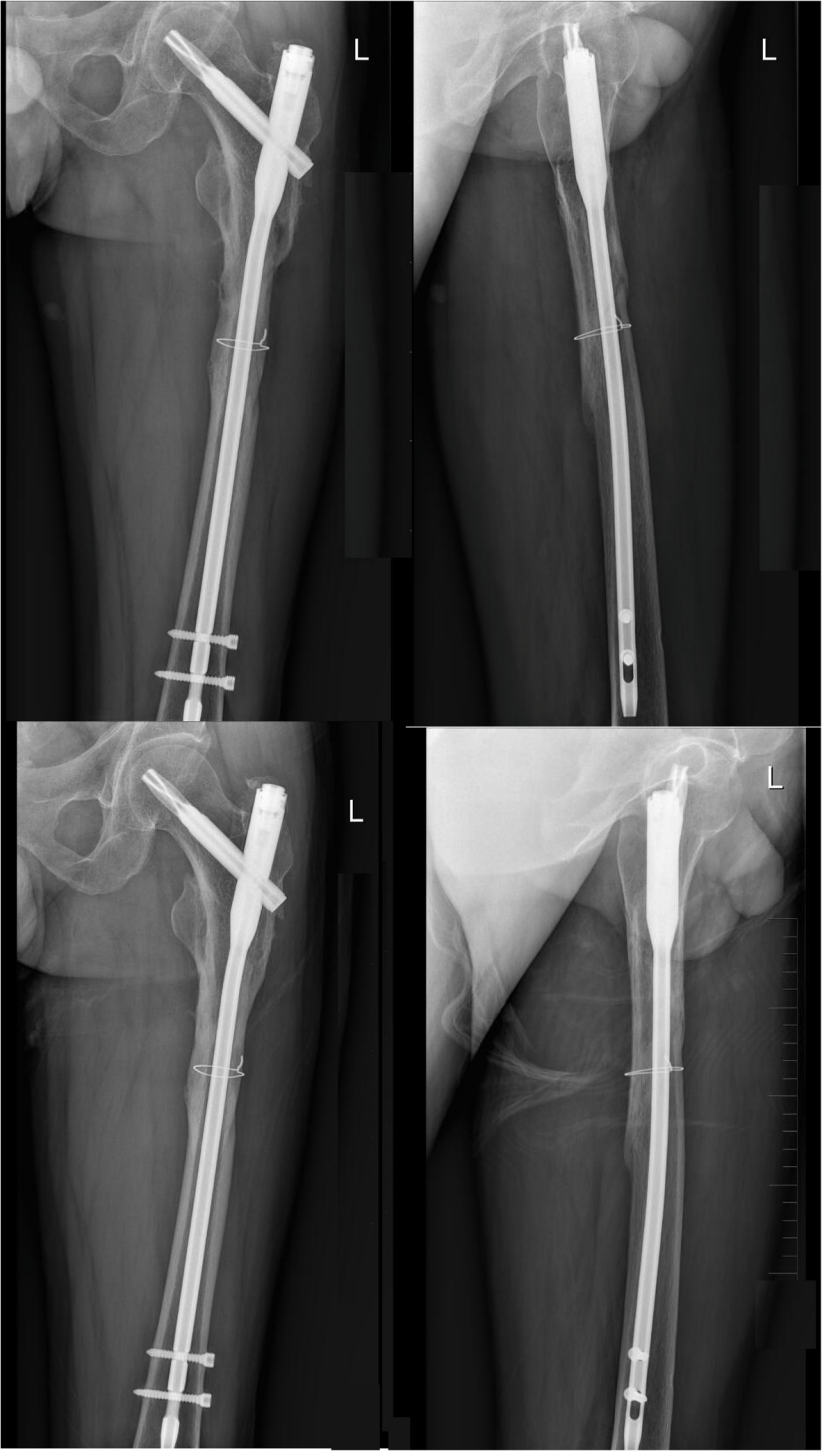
Re-examination 6 months after the operation showed obvious callus formation and fuzzy fracture line (upper). The re-examination 12 months after the operation indicated that the fracture end alignment was good and the fracture had been completely healed. a, b Preoperative X-ray of the affected hip joint (lower)
